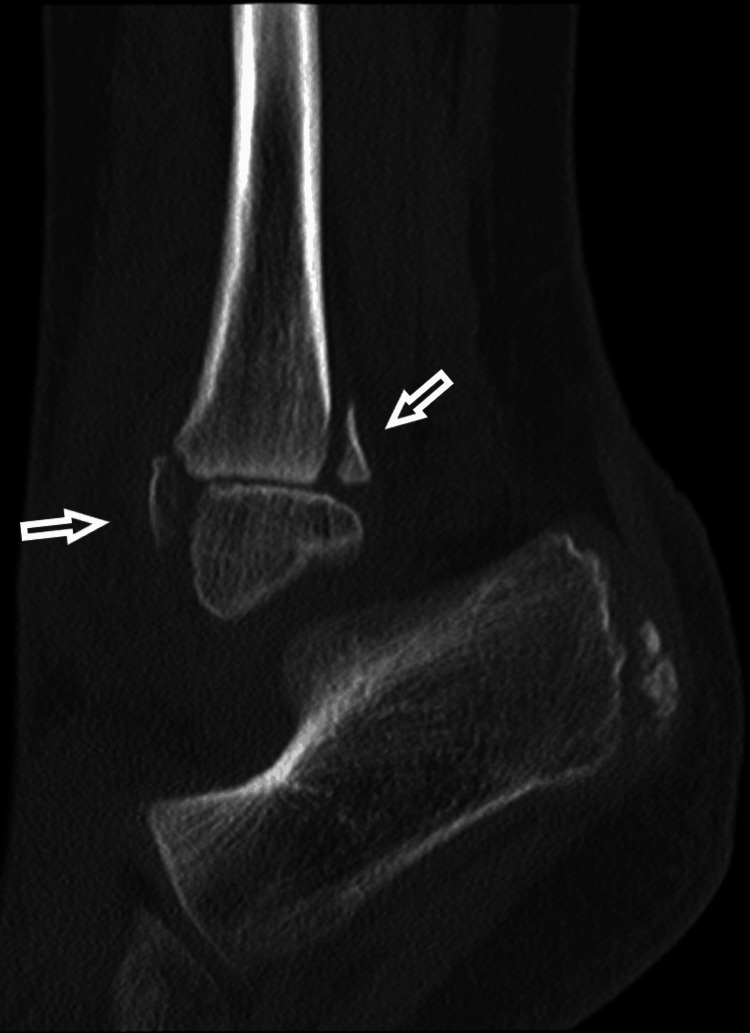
A lateral projection of the CT scan, with the arrows pointing to the metaphyseal fragments of the distal fibular epiphysiolisthesis.
